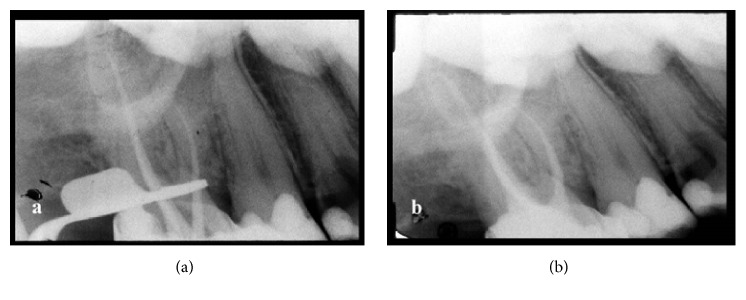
(a) Radiograph with the gutta-percha points: case report number 1. (b) Final radiograph: case report number 1.
